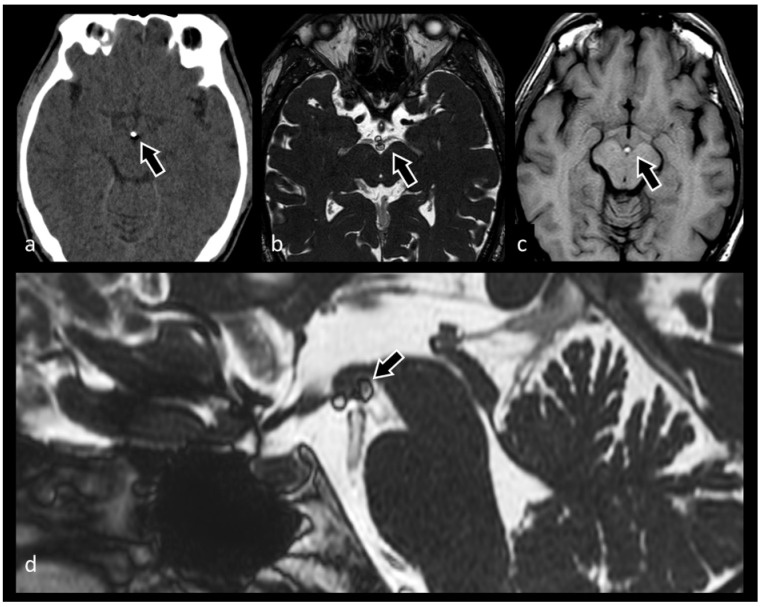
Axial brain CT (a) shows two incidentally-discovered focal calcified formations (arrow) located in the interpeduncular cistern, indissociable from the basilar artery. Axial and sagittal 3D CISS images (b,d) characterize the formations (arrow) and their relationship with the basilar artery well, and show the calcifications are mainly located at the periphery of the lesions, appearing as subtle hypointense rims. MRI also reveals the lesions (arrow) present a lipidic core, which appears hyperintense on spin-echo T1-w. sequence (c) and shows signal dropout on fat-suppressed images (not shown). Findings were consistent with osteolipomas.
